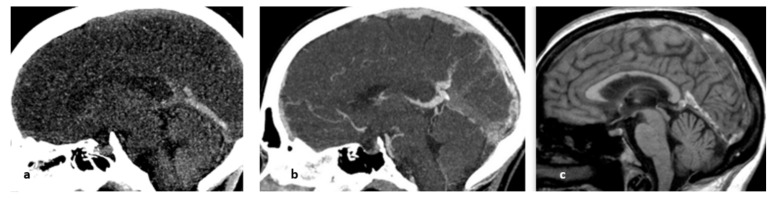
From left to right: (a) nonenhanced CT, sagittal plane; (spontaneous hyperdensity of the straight sinus); (b) after the injection of iodinated contrast media, absence of opacification of the straight sinus, thrombosis of the superior sagittal sinus above the torcular Herophili; (c) MRI, nonenhanced sagittal T1 sequence: spontaneous hypersignal of the thrombus.
